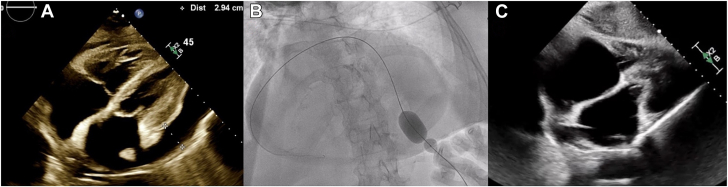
Subxiphoid transthoracic echocardiogram before the procedure (A), percutaneous balloon pericardiotomy (B) and echocardiogram after (C) the procedure of patient 4. Note the marked improvement in pericardial effusion posterior to the left ventricle.
